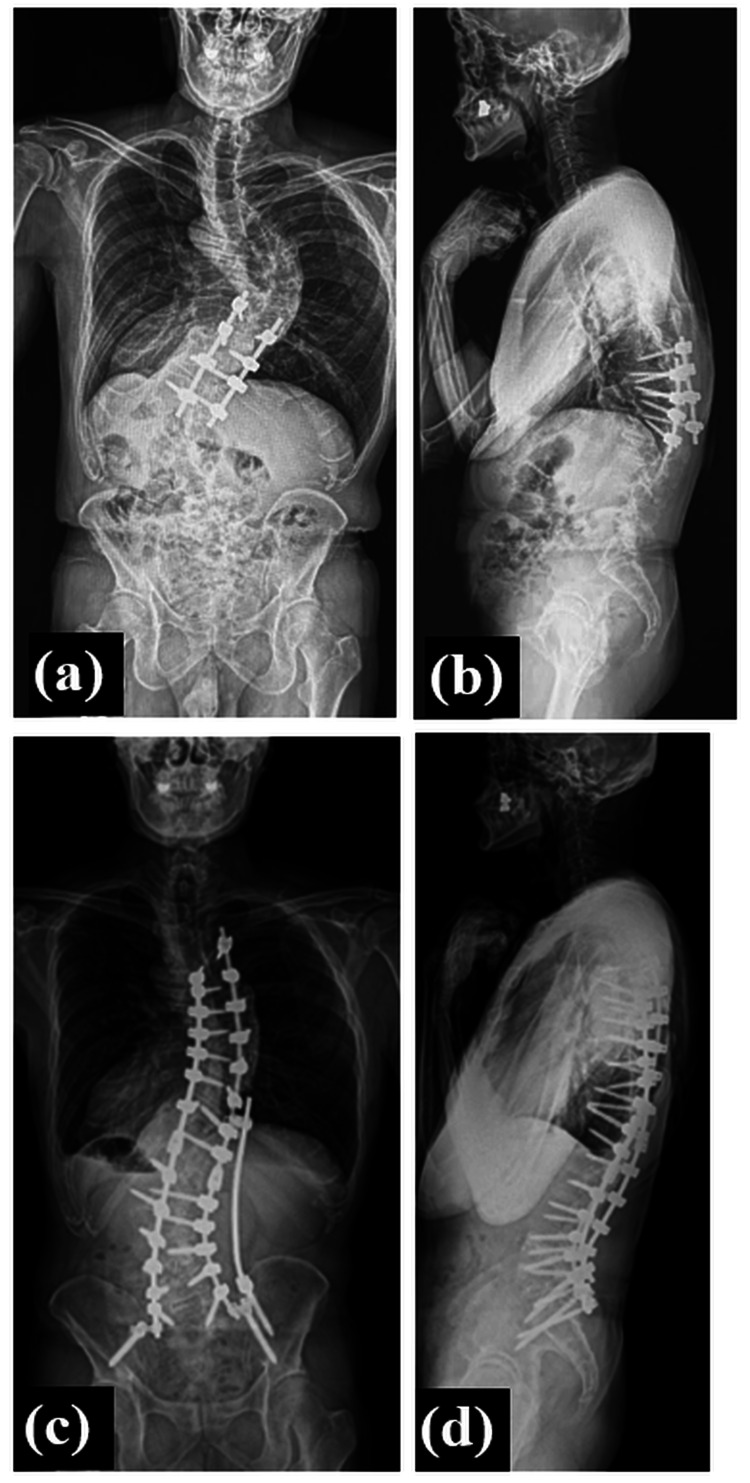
Postoperative radiographs Postoperative standing posteroanterior radiographs obtained one year after the first surgery (a, b) and at the final follow-up after the second surgery (c, d).
